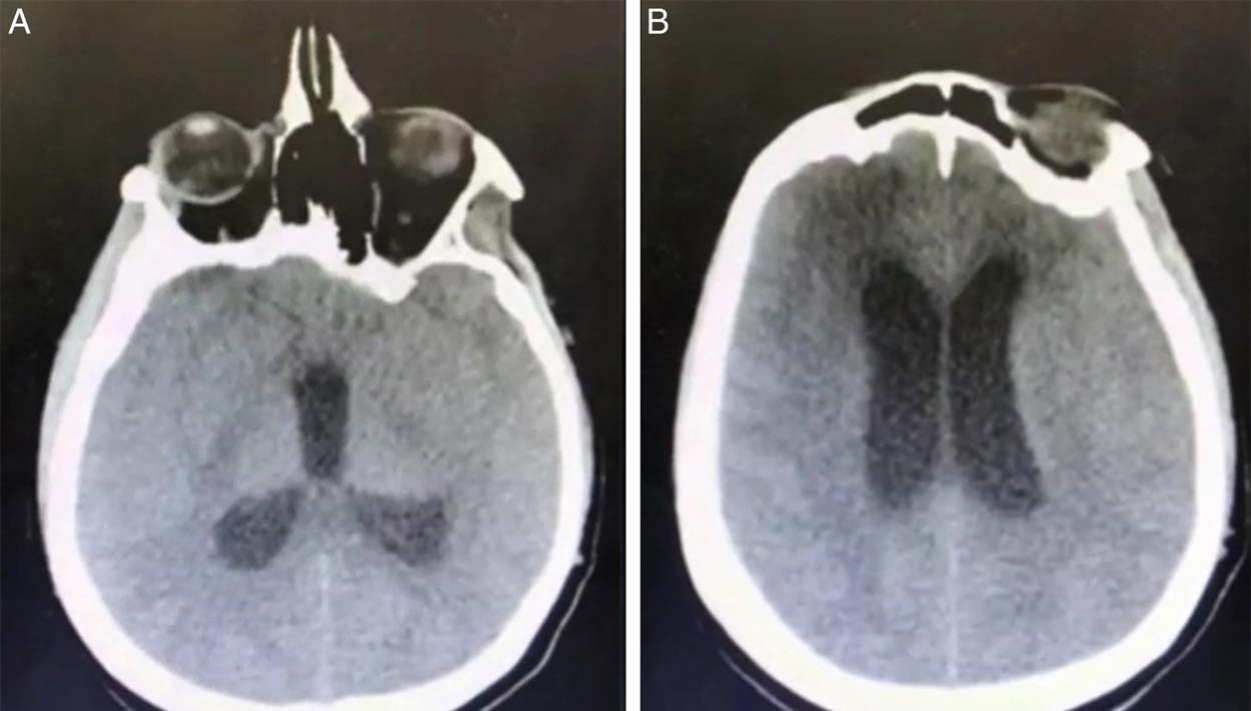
(Colored). A. CT, axial section, dilation of the 3rd ventricle indicating hydrocephalus. B. CT, axial section, dilation of the lateral ventricles indicating hydrocephalus.
